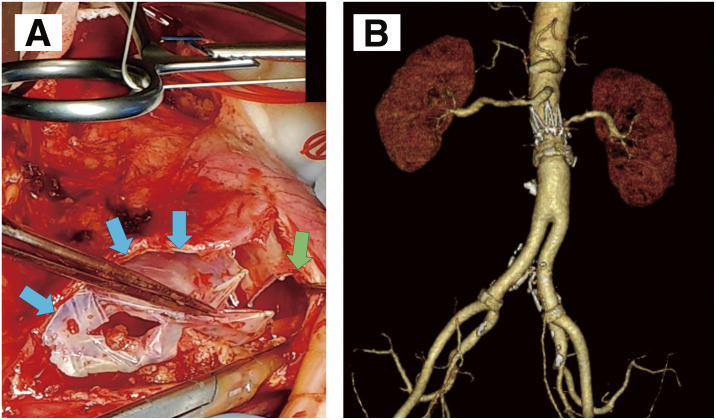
(A) Intraoperative photograph after midline laparotomy and opening of the aneurysm sac. Complete disconnection is confirmed at the modular junction between the AFX2 main body and the VELA suprarenal extension (blue arrow: AFX2 main body; green arrow: VELA). (B) Three-dimensional reconstruction of contrast-enhanced computed tomography following open graft replacement, showing partial preservation of the VELA suprarenal extension graft.
